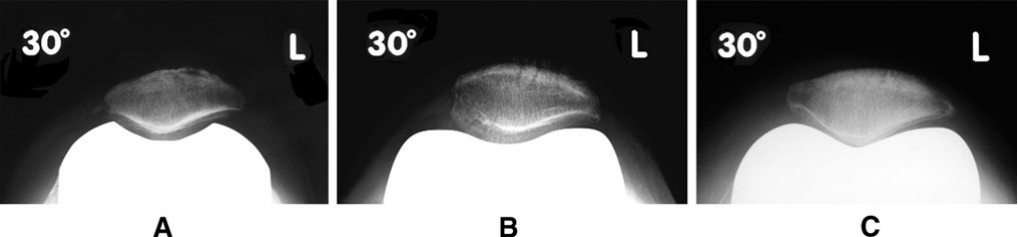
Post-operative skyline radiographs showing the native patella articulating with three different prosthetic femoral TKA components displaying varying degrees of ‘patella-friendly’ design features. A: Optetrak^®^, Exactech, USA; B: AGC^®^ Biomet, USA; C: LCS^®^, DePuy, USA
